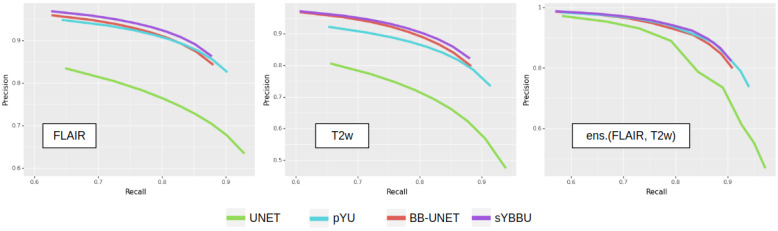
Mean precision-recall graphs of the different proposed segmentations on the TCGA-GBM${}^{test}$ dataset. To focus on the most interesting part of the plot, we only plotted the precision-recall scores for thresholds between 0.1 and 0.9. From the left to the right, Using the FLAIR, using the T2w, and ens.(FLAIR, T2w).
